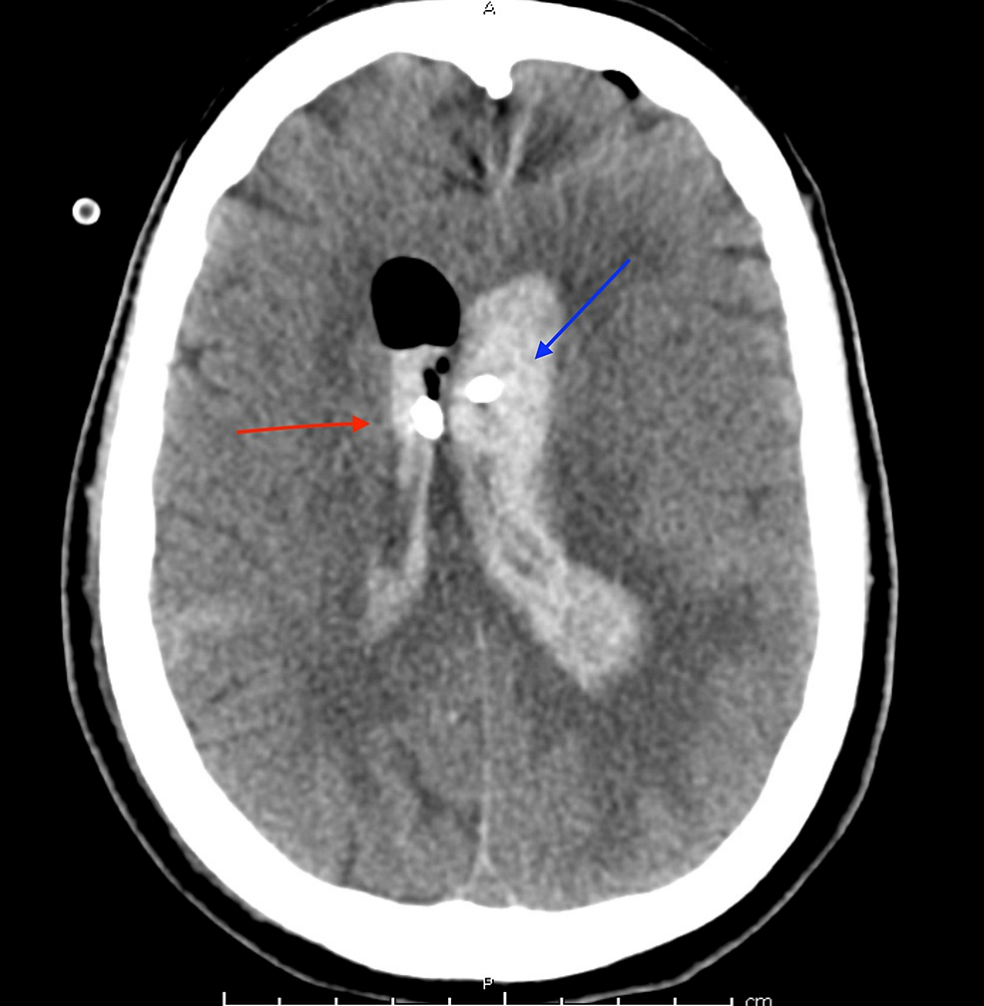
Head CT demonstrating right-sided IRRAflow catheter (red arrow) and left-sided standard catheter (blue arrow)
